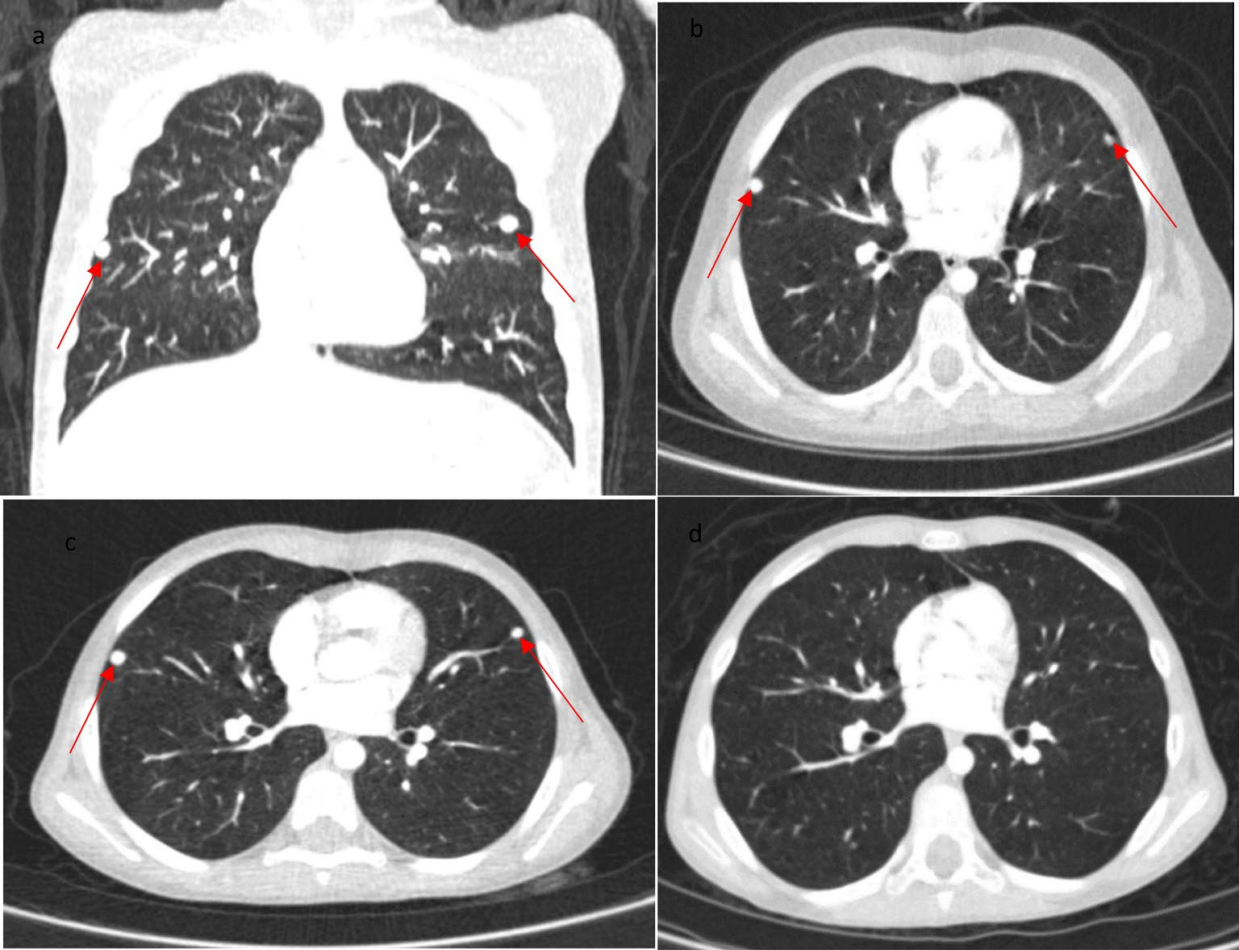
Contrast‐enhanced CT scan of the patient in June 2022, 3 months after initiating pazopanib, demonstrated bilateral multiple pulmonary nodules in coronal (a) and axial views (b) (arrows). CT scan was performed in February 2023, 6 months after initiating pazopanib, showing stable pulmonary nodules (c) (arrows). CT scan of the patient in July 2024, 6 months after initiation of ICI therapy, revealed no pulmonary nodule (d).
